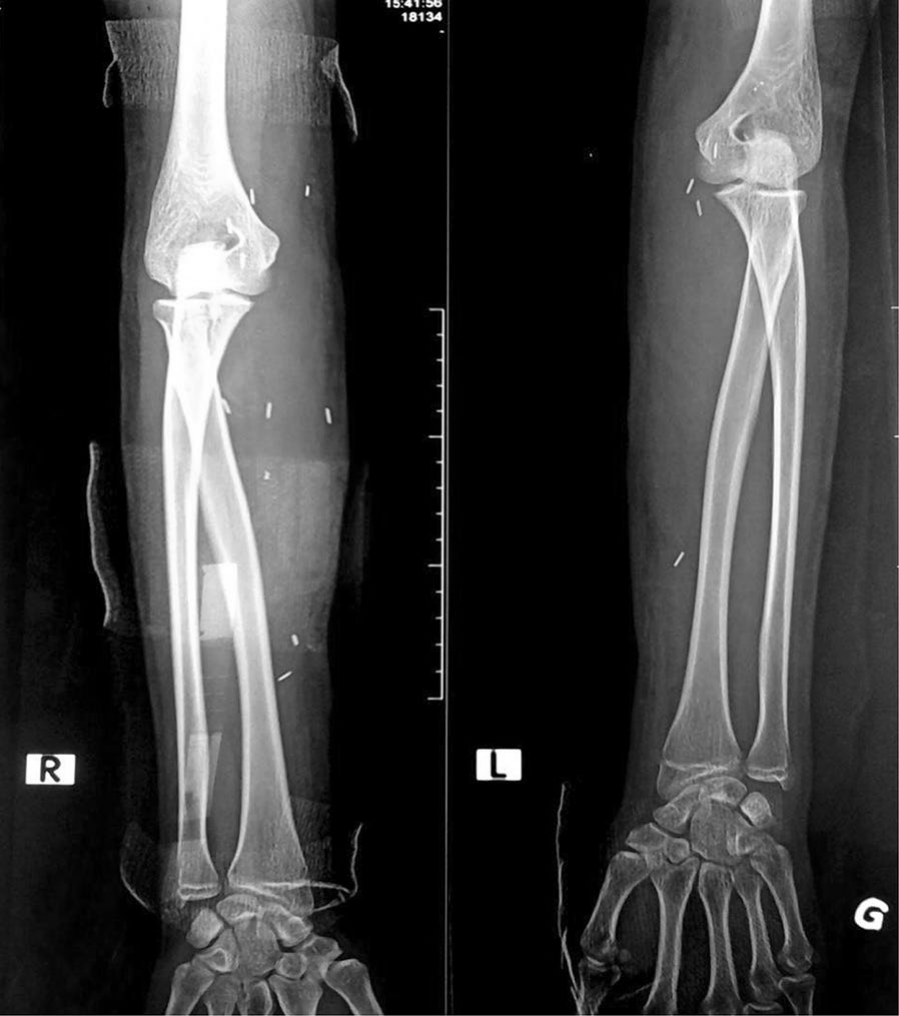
post-surgical x-rays of hands showing full clearance of mercury. (Plaster casts and surgical clips also visible in this x-rays)
